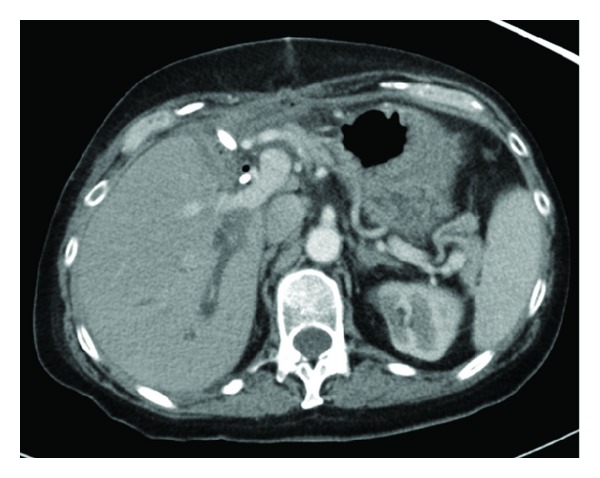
Isolated dilated right posterior sectoral duct. CT scan demonstrating dilated right posterior duct after adequate drainage of the biloma. Note the anterior ductal system is decompressed.
